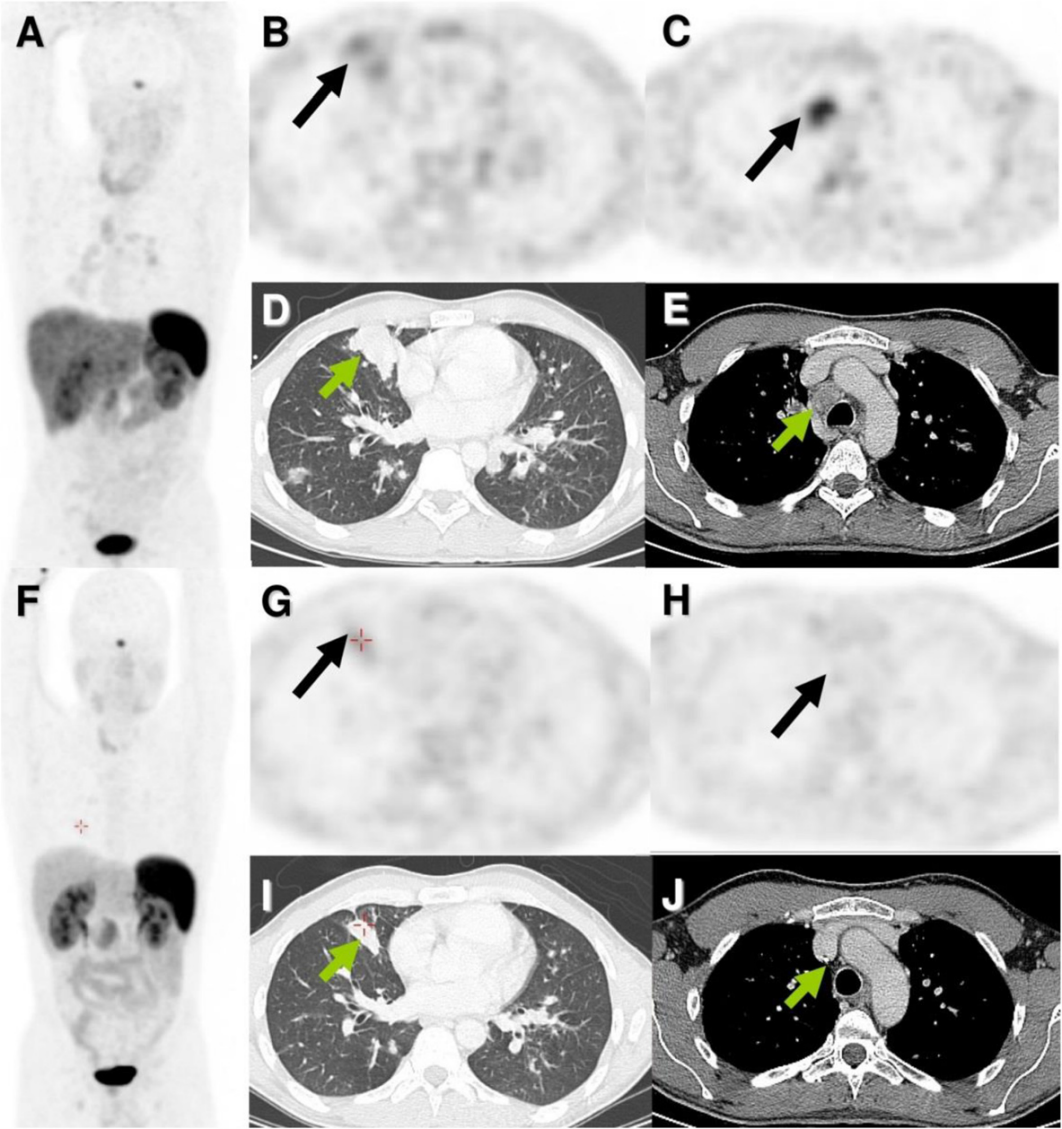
Patient with initially diffuse pulmonary neuroendocrine cell hyperplasia (DIPNECH) with transformation into an atypical carcinoid was referred for Ga-68 DOTATOC PET/CT. Based on weak somatostatin receptor expression, patients was treated with chemotherapy (folinic acid, 5 fluorouracil, and oxaliplatin) and showed a good response. a-e Before chemotherapy. f-j After chemotherapy. a, f Maximum intensity projection PET images. b, c, g, h Axial PET images. d, e, i, j Corresponding axial CT images. Partial remission of the mildly receptor positive lesion in the right lung is well appreciated on CT (*arrows*). On MIP images, the previously receptor-positive hilar and mediastinal lymph node lesions also show response to treatment
